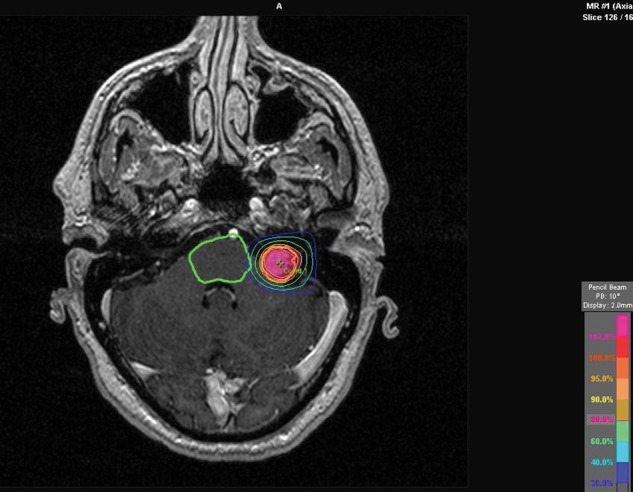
MRI-based target definition, 100% dose at reference point.
